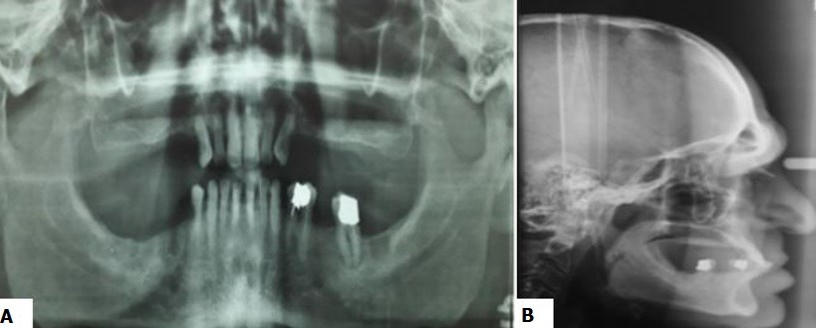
X-Ray examination; (A) orthopantomogram examination; (B) cephalogram examination
